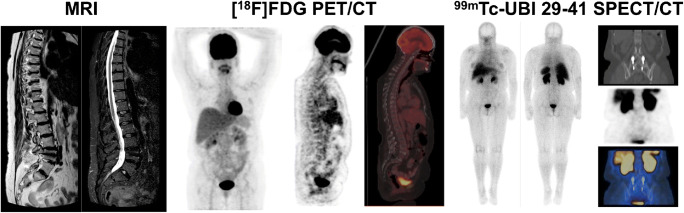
A 52-year-old woman previously submitted to lumbar surgery L4-S1 presented with sensory loss in the L2-L3 region; serum CRP was mildly increased, ESR was normal. On MRI (left) the sagittal T2WI and STIR images of the thoracolumbar spine demonstrate posterior spinal implant at L4 with no MRI features to suggest postoperative spondylodiscitis. No increased tracer uptake was noted on [^18^F]FDG PET CT images (middle) nor on ^99m^Tc-UBI 29-41 SPECT/CT (right). Final diagnosis of this patient at the final 7-month follow-up was “no postoperative spine infection”
